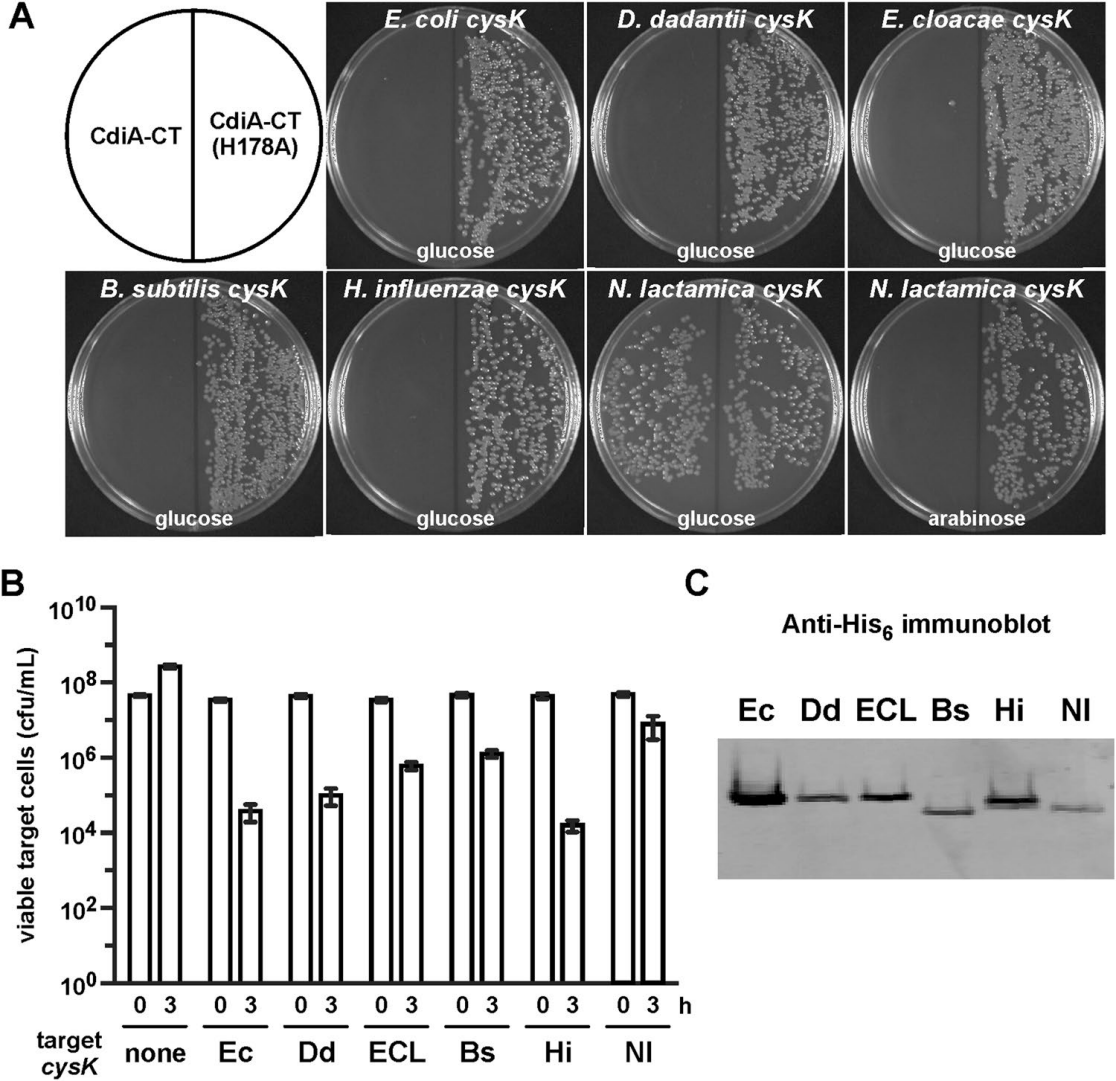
Heterologous CysK promotes CdiA-CT toxicity. (A) Activation of internally expressed CdiA-CT toxin. Plasmids encoding CdiA-CT and CysK proteins were introduced into *E. coli ∆cysK* cells, and transformants isolated on selective media supplemented with D-glucose or L-arabinose as indicated. To control for transformation efficiency, a plasmid encoding catalytically inactive CdiA-CT(H178A) was also tested. (B) CDI competition co-cultures. Inhibitor cells that deploy CdiA-CT were incubated with *E. coli ∆cysK* target bacteria that express CysK-His_6_ from the indicated bacterial species. Viable target bacteria were quantified as colony forming units per mL upon mixing and after 3 h of co-culture. Presented data are averages ± standard errors for four independent experiments. (C) Immunoblot analysis of heterologous CysK-His_6_. Total protein was isolated from the target-cell strains in panel B and analyzed by immunoblotting using antibodies to the His_6_ epitope. 10 μg of total protein was loaded in each lane.
